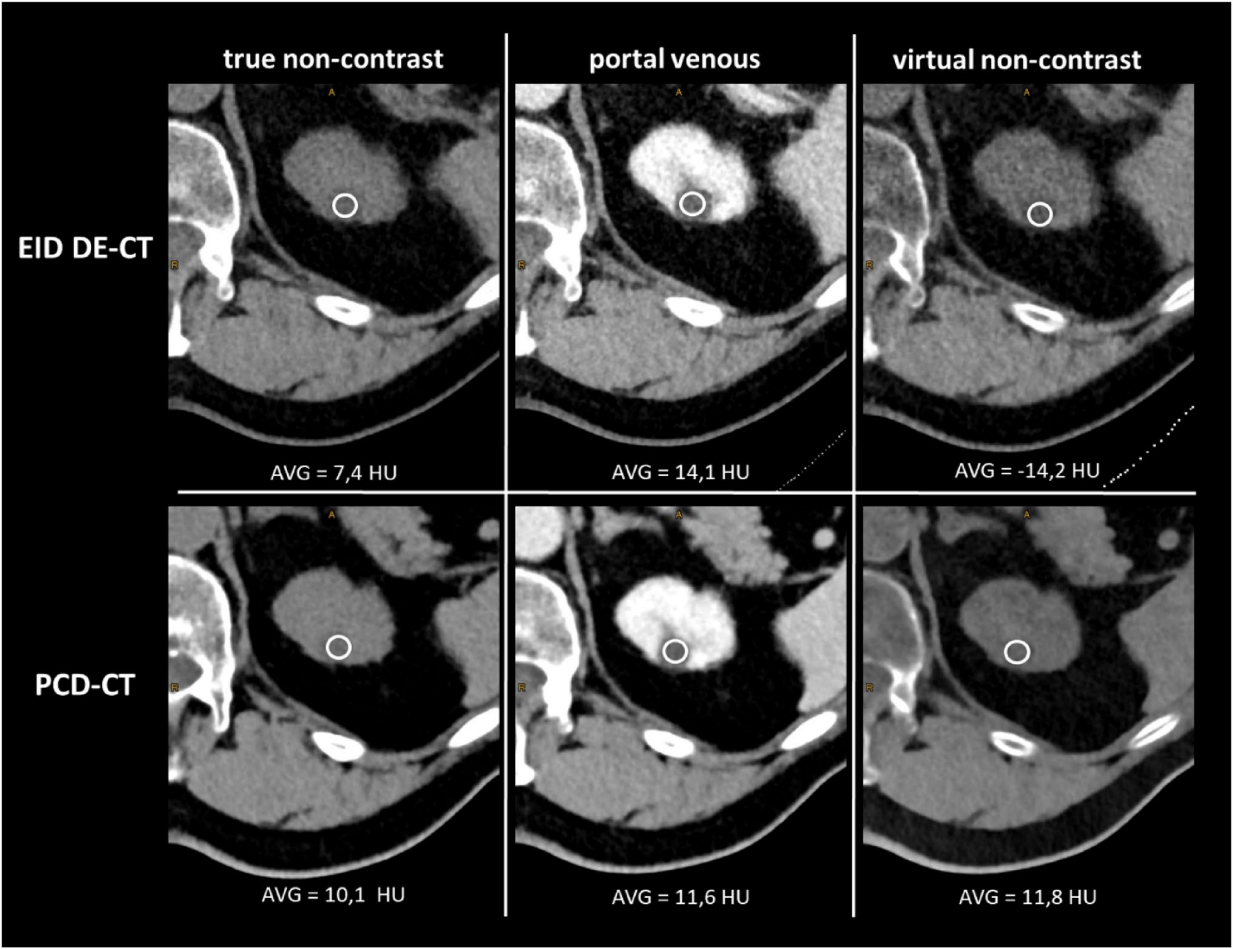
Example of Density-measurements in a simple renal cyst on EID DE-CT and PCD-CT images using true non-contrast, portal venous and virtual non-contrast derived images (from left to right). While true enhancement is < 20 HU, EID DE-CT VNC-derived values are remarkably low leading to false-positive enhancement > 20 HU. PCD-CT = photon-counting detector CT; DE EID-CT = dual-energy integrating detector CT
